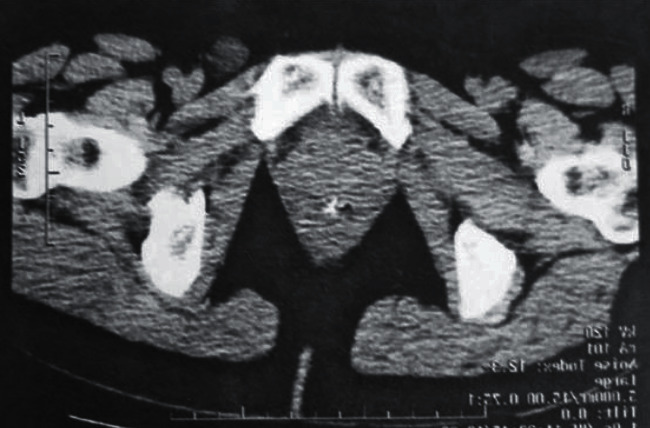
Computed tomography: in the inguinal region, a clearly demarcated formation diameter of 2 cm, with solid and cystic elements, is showed (swollen lymph node?) (arrow).
